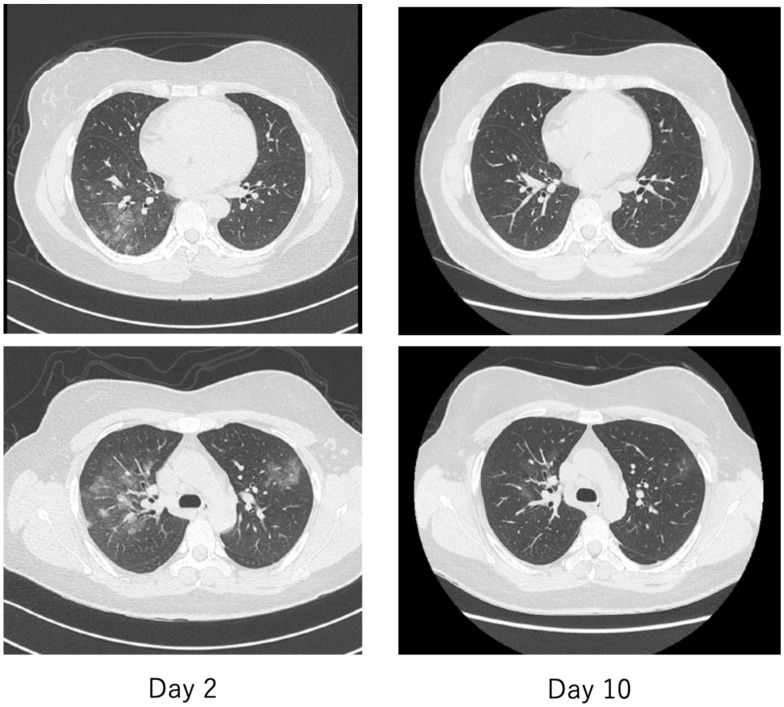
Chest computed tomography (CT) of a patient with mild COVID-19, the same patient as in Figure 12. On the second day, there were multiple faint frosted shadows bilaterally (left), but by the tenth day, the abnormal shadows had almost disappeared (right).
